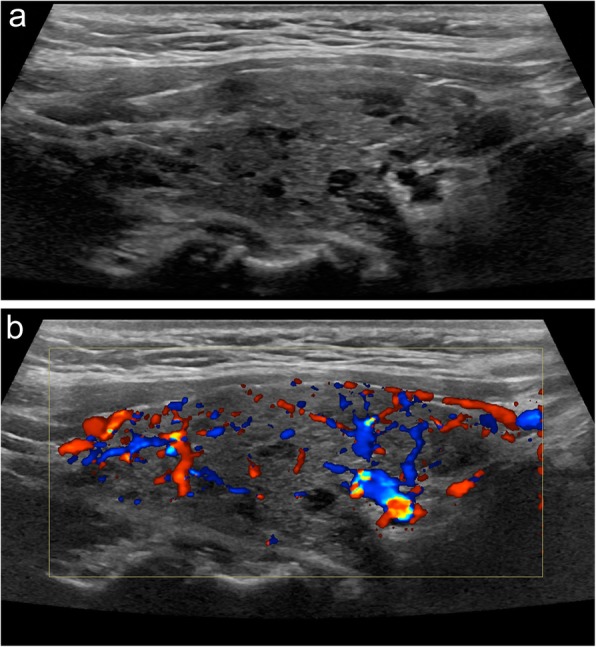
Dilated thoracic duct. Axial (a) and coronal (b) contrast CT images demonstrating dilated thoracic duct (arrow) draining into the confluence of the left subclavian and left internal jugular veins. This is considered a normal variant and is important to not mistake it for a pathologic lesion
